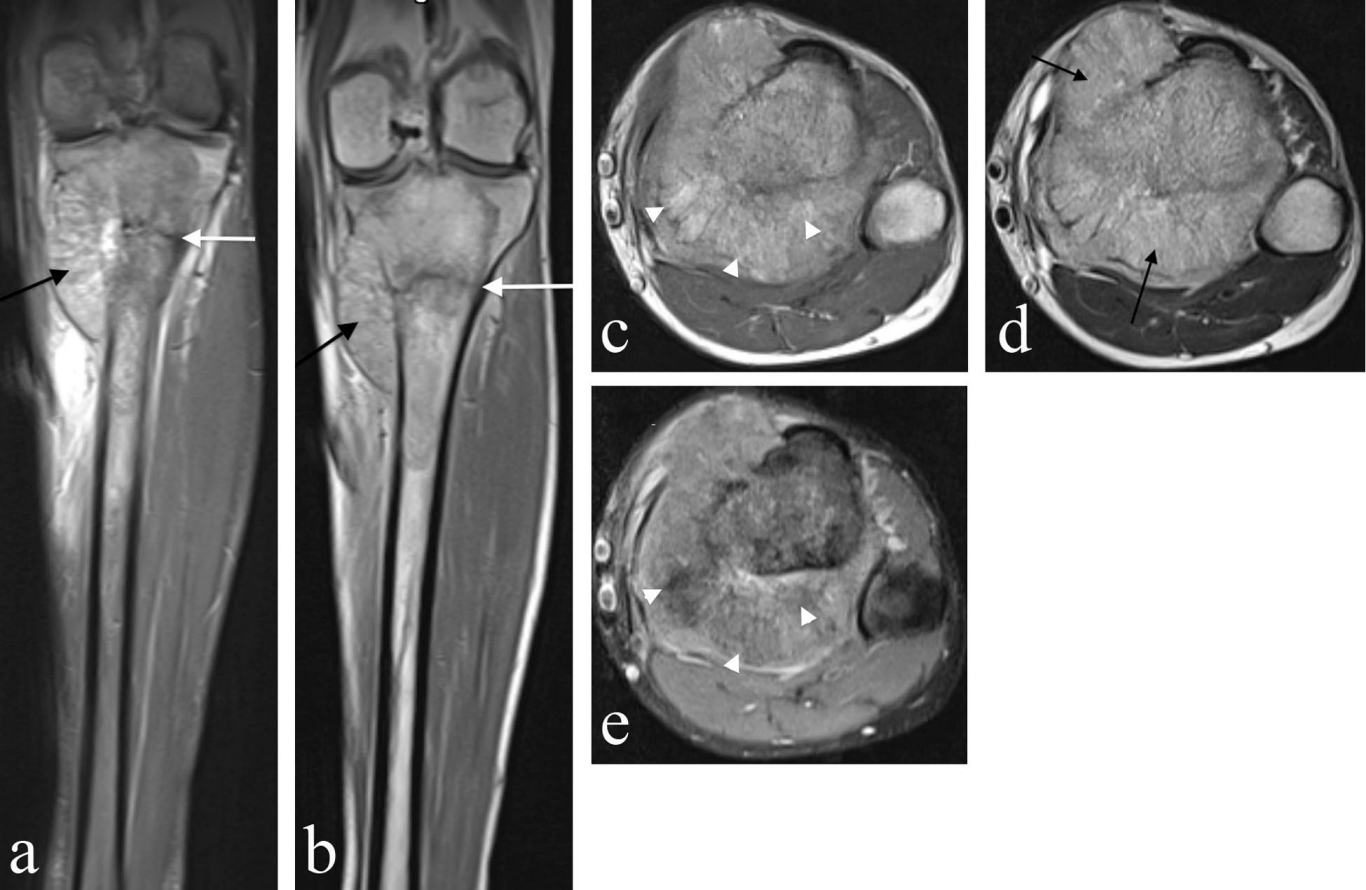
Magnetic Resonance Imaging (MRI) of the proximal tibial lesion. a Coronal STIR, b coronal T1-, c axial T1-, d axial T2-weighted and, e axial proton density with fat saturation. The images demonstrate an ill-defined proximal tibial medullary based lesion extending to the subarticular surface. There is extensive cortical destruction with an almost circumferential soft tissue mass, which has a spiculated appearance (black arrows) displacing the posterior muscles and popliteal neurovascular bundle. Tumour breaches the deep fascia anteriorly to extend into the subcutaneous tissues and penetrates the interosseous membrane to extend around the anterolateral tibial cortex. Heterogeneous partly high T1 signal suppresses with fat saturation sequences supporting a partly fatty matrix (white arrowheads). The pathological fracture is clearly seen extending transversely across the proximal tibia (white arrows)
